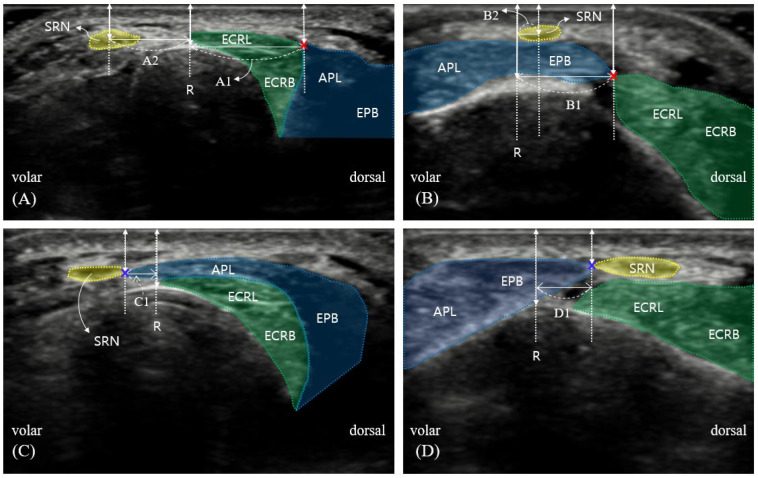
Representative ultrasonographic images of the wrist at the four designated levels (A–D): (A) Level A, (B) Level B, (C) Level C and (D) Level D. The red ‘X’ marks denote the intersection points at Levels A and B, while the blue ‘X’ marks indicate the SRN crossing points at Levels C and D. A1, A2, B1, B2, C1, and D1 indicate the horizontal distances from the superficial radial border to each respective landmark. Vertical white arrows indicate the depths from the skin surface to the SRN, intersection/crossing points, and the radius. R: radius; APL: abductor pollicis longus; EPB: extensor pollicis brevis; ECRL: extensor carpi radialis longus; and ECRB: extensor carpi radialis brevis.
